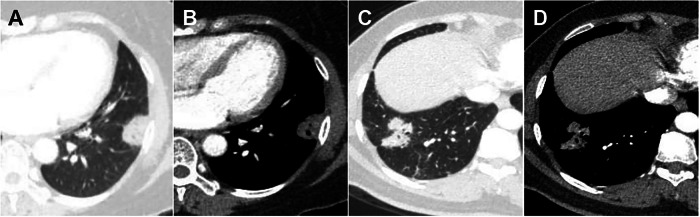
A, B Images in a 59-year-old woman with primary LMA in clinical stage I. Axial contrast-enhanced CT image shows a 29-mm well-defined lobulated pure-solid nodule with an air bronchogram sign in the left lower lobe. The CT attenuation of the lesion was 20 HU. C, D Images in a 55-year-old woman with primary LMA in clinical stage I. Axial contrast-enhanced CT image shows a 39-mm well-defined lobulated pure-solid nodule with an air bronchogram sign in the right lower lobe. The CT attenuation of the lesion was 22Hu. Genetic analysis indicated that these two patients carry KRAS mutation, and there was no recurrence or death within 5 years after surgery
